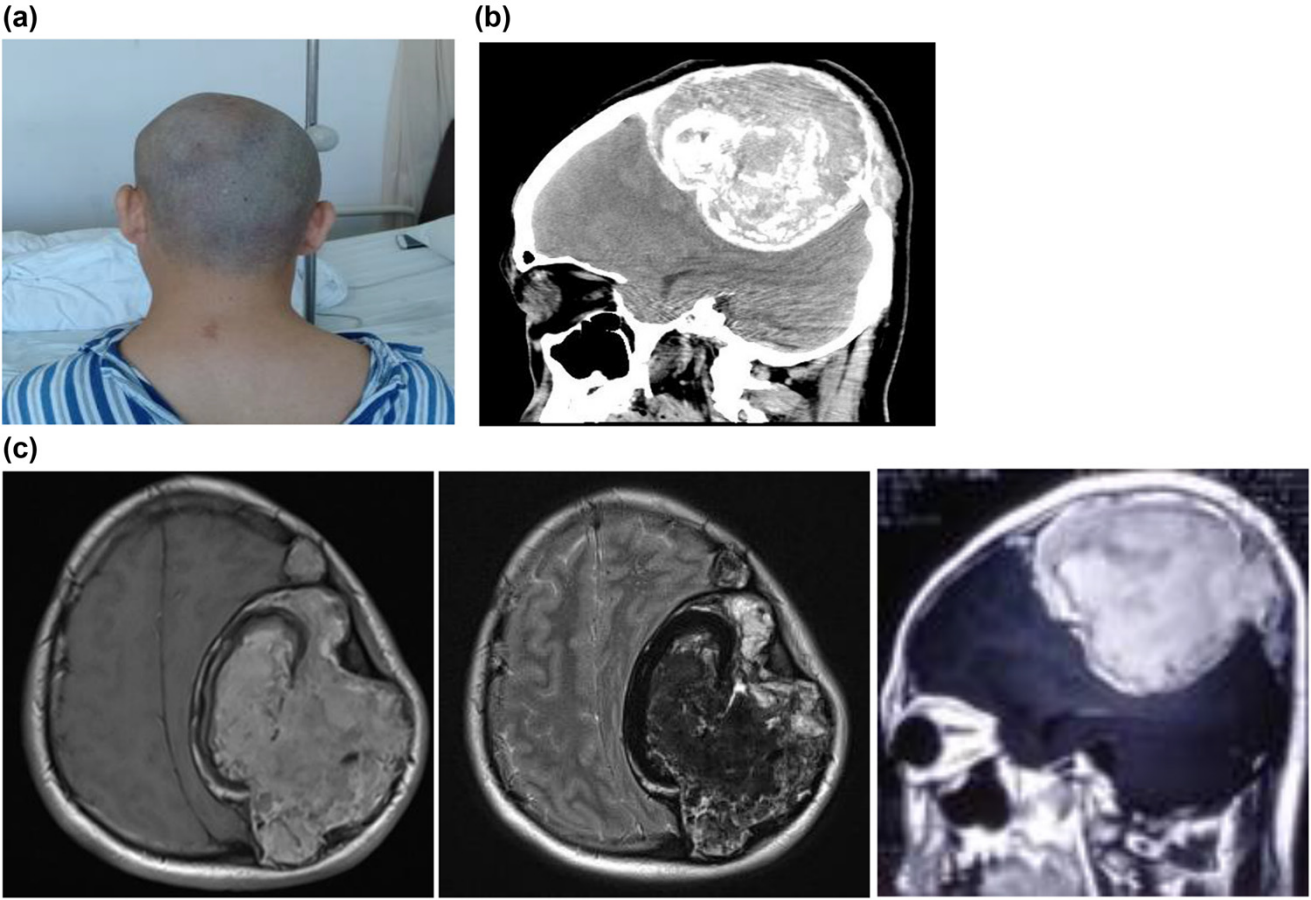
Lesion examination before surgery. (a) Lesion appearance. A bulge on the left frontal-temporal parietal bone was observed, with asymmetry on the left and right sides. (b) Preoperative head CT examination. Abnormal density lesions on the left frontal-temporal parietal bone, with multiple irregular calcifications and clear boundaries were observed. (c) Preoperative MR examination. MRI T1WI: the left frontal lesion showed a slightly higher signal. MRI T2WI: mixed signal. MRI enhanced: visible lesion enhancement.
